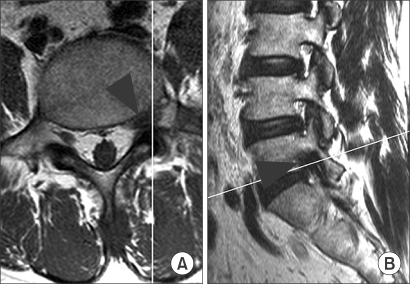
The axial (A) and sagittal (B) MR images of a 58 year-old man are shown. The white line in each figure indicates the sectioning plane for the other figure. A foraminal disc herniation on the left side at L5-S1 (arrowheads) was interpreted as positive for lateral disc herniation by all three observers during both image-reading sessions.
